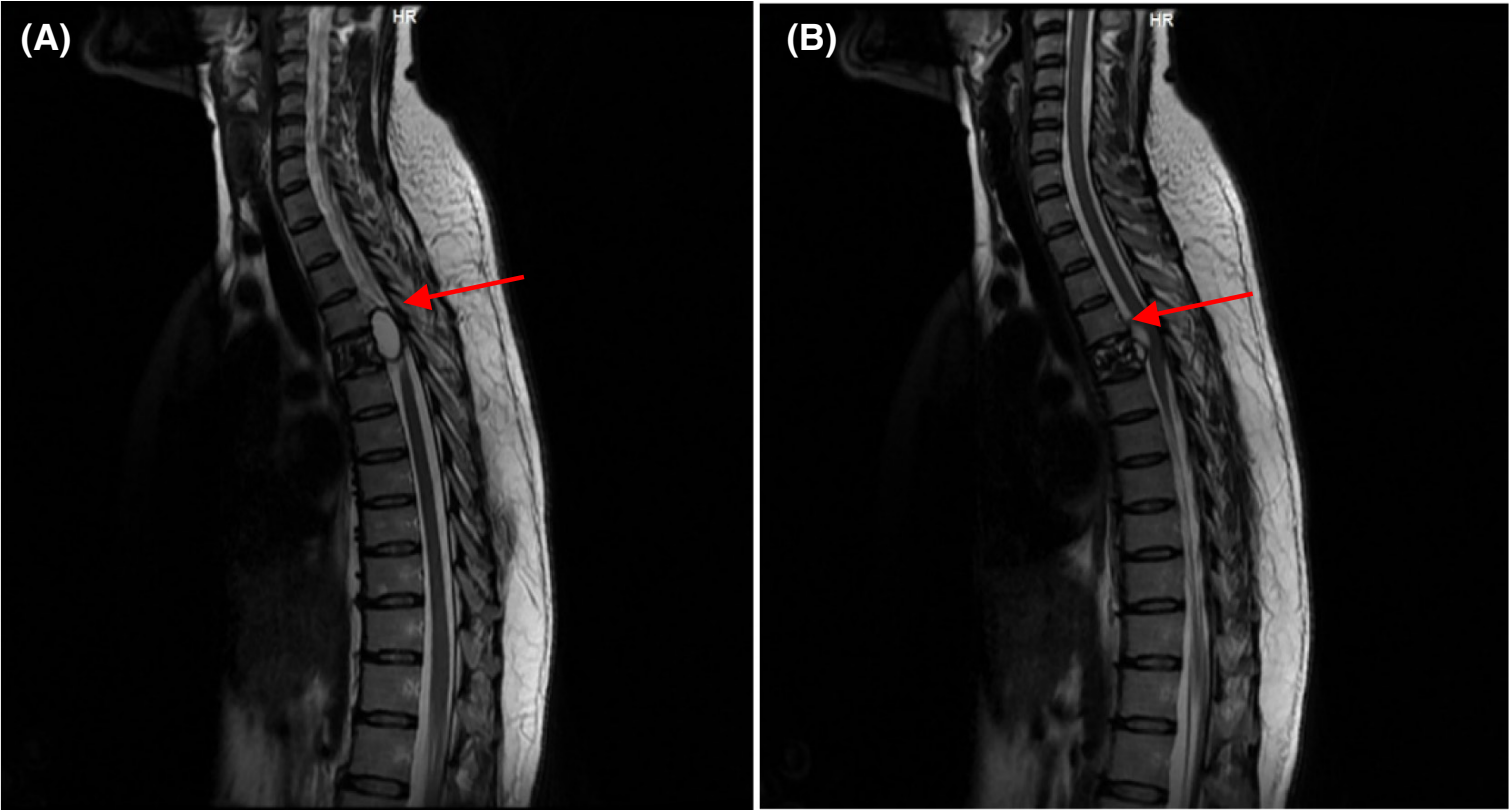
Magnetic resonance imaging (MRI) of the thoracic spine: (A) Hydatid cyst and (B) vertebral fracture.
